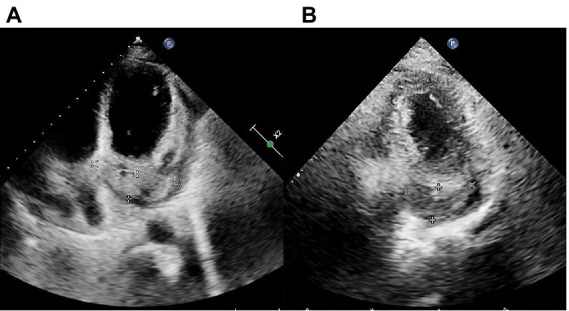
Transthoracic echocardiography (TTE) findings pre- and post-chemotherapy. (A) Pre-chemotherapy TTE revealing filling of the coronary sinus with inhomogeneous echogenic material measuring approximately 21.4 mm × 68.3 mm. (B) TTE after the second cycle of chemotherapy showing filling of the coronary sinus with inhomogeneous echogenic material measuring approximately 22.5 mm × 43.4 mm are shown.
